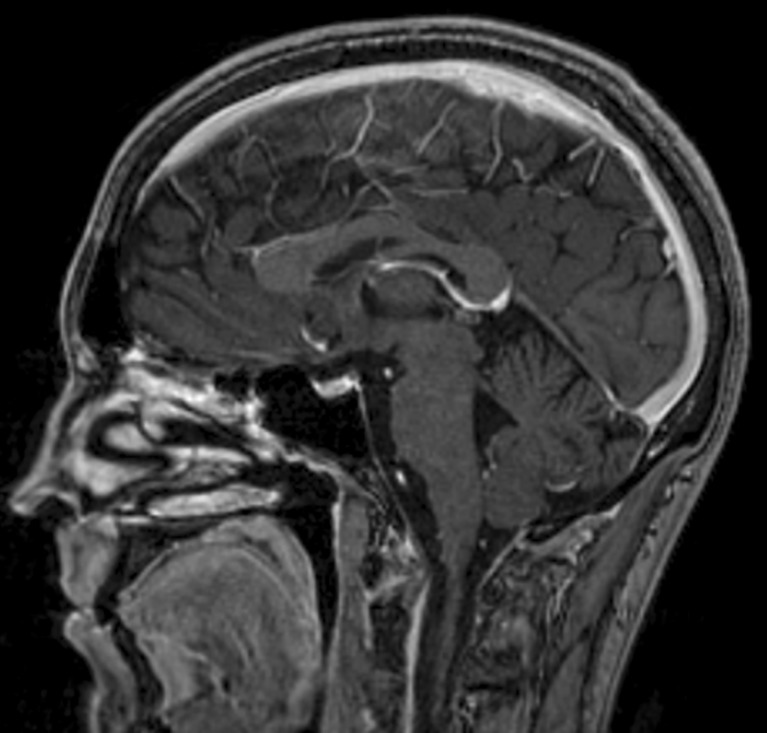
MRI after 2 months of treatment with apixaban, showing resolution of superior sagittal sinus thrombosis
